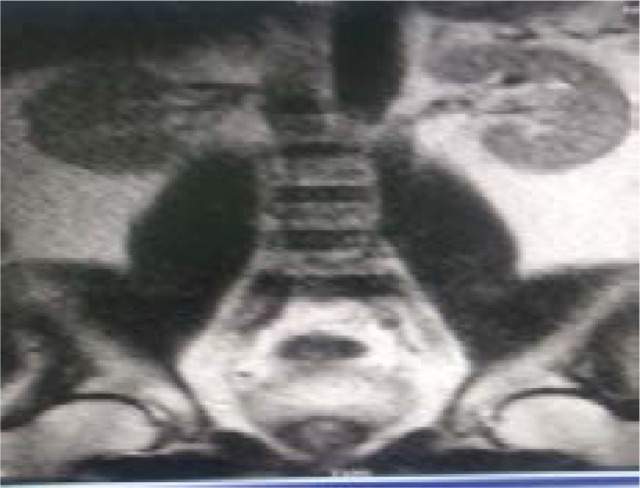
Chemical shift artifact seen on lumbar spine MRI
